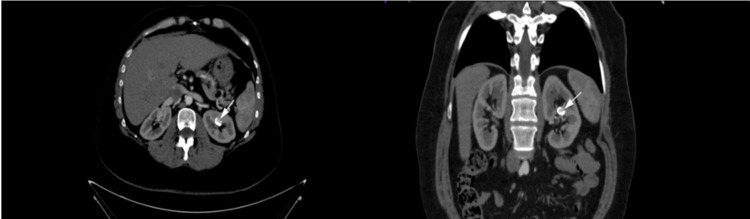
Contrast-enhanced CT (CECT) in the artery phase reveals significant findings in both axial (a) and oblique coronal-plane reconstructions (b). Notably, there is a renal artery saccular aneurysm, indicated by the arrows, which presents as a focal dilation and is engorged with contrast material, characteristic of a saccular aneurysm but with multiple parietal calcifications. This imaging modality provides a detailed visualization of the arterial vasculature, facilitating the precise identification and characterization of pathological conditions such as aneurysms. This figure is the original work of the authors. Patient consent for the use of the image was obtained, as mentioned in the patient consent form (point no. 12).
